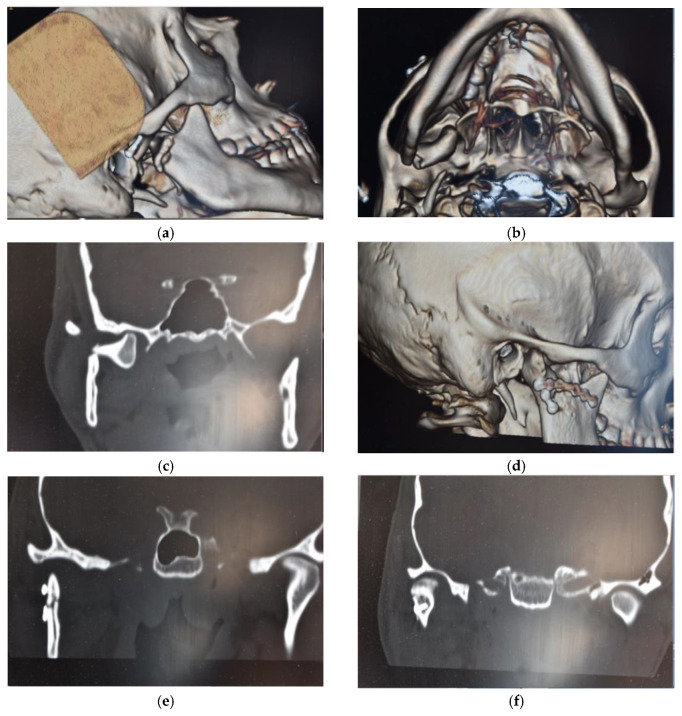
(a,b) Pre-operatory volumetric CT; (c) pre-operatory coronal CT; (d) post-operatory volumetric CT; and (e,f) post-operatory coronal CT of patient #4.
